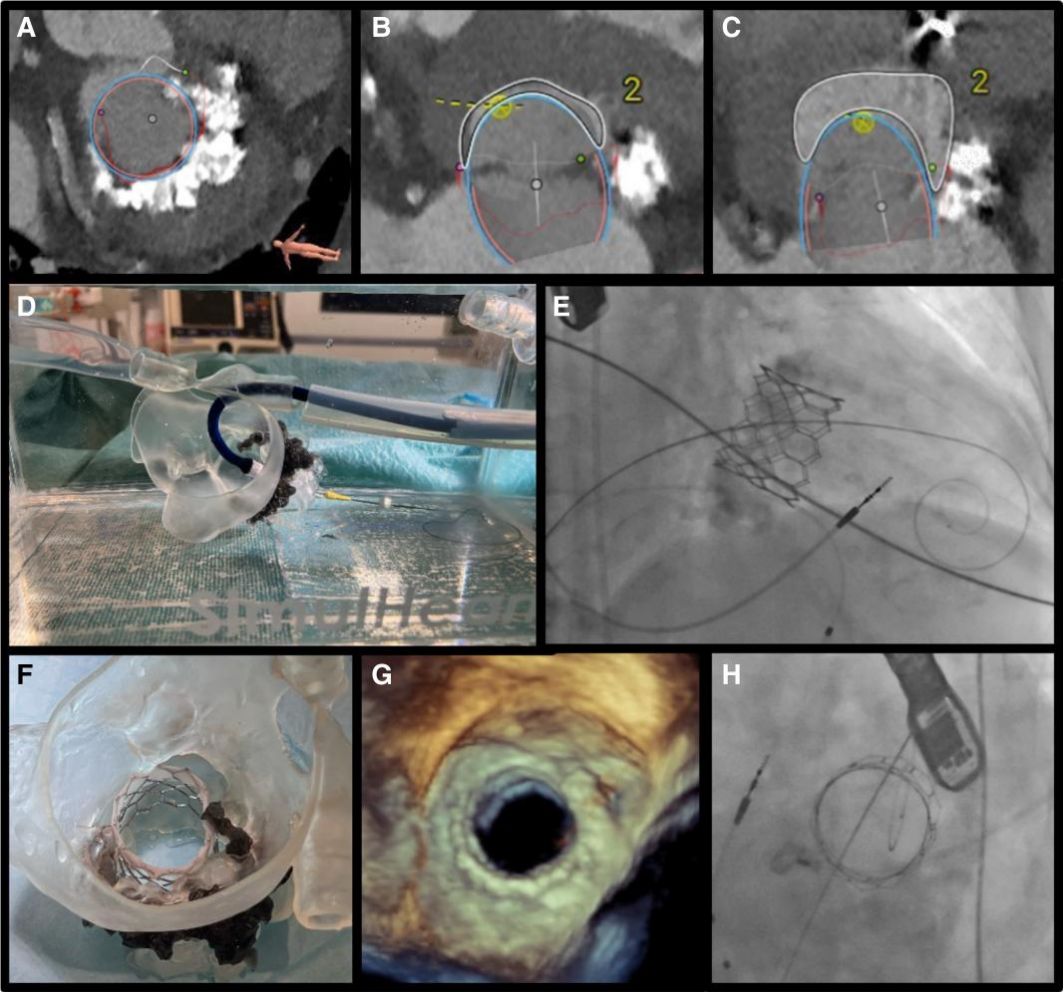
An 81-year-old woman with heart failure with normal ejection fraction was diagnosed with mitral annular calcification (MAC) causing moderate–severe mitral disease. Heart team decision was to evaluate candidacy for a valve-in-MAC (ViMAC) procedure due to high anatomic and surgical risk (STS score 12.9%, Euroscore II 5.36%). Pre-procedural CT showed feasibility for ViMAC based on mitral annular area and a MAC score of 8 (*Panel A*), but predicted a significant risk of left ventricular outflow tract (LVOT) obstruction with a neoLVOT area of 129 mm^2^ (*Panel B*). To address this, alcohol septal ablation (ASA) was performed. Follow-up CT showed a substantial increase in neoLVOT area to 411 mm^2^, strikingly reducing the obstruction risk (*Panel C*).
